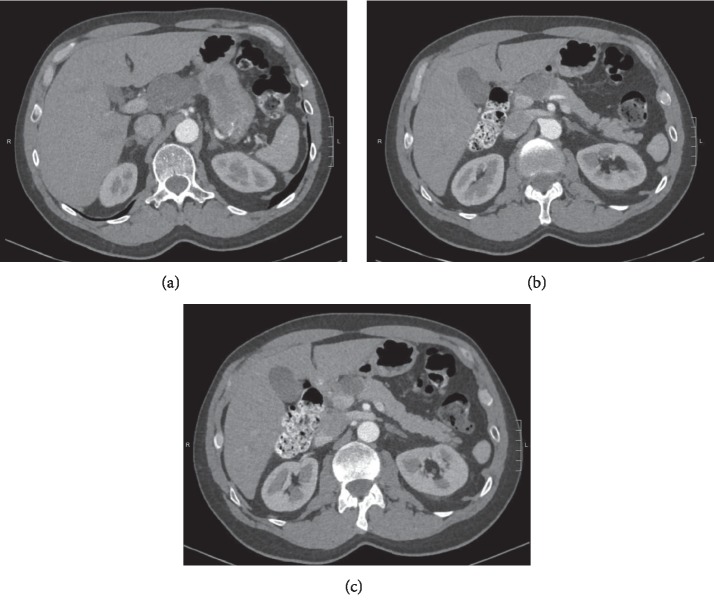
Series of images (proximal to distal) from abdominal CT showing a 36 × 52 mm mass in the pancreatic head with no pancreatic ductal dilation.
